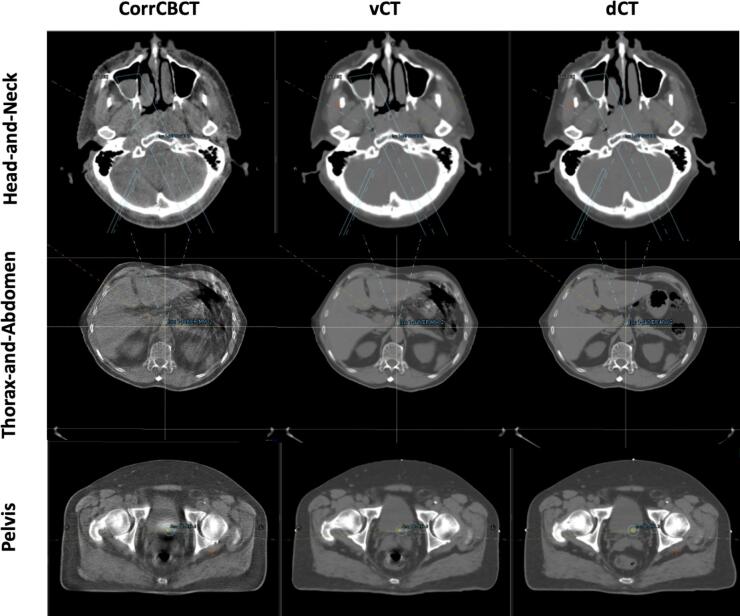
Comparison of corrected CBCT (corrCBCT), virtual CT (vCT), and deformed reference CT (dCT) images for the three treatment sites.
